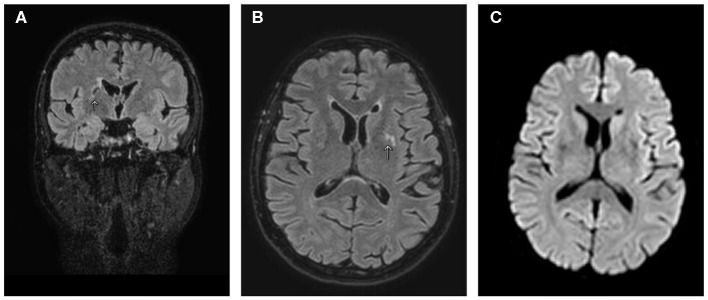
MRI of the brain after 3 months of follow-up. T2/fluid-attenuated inversion recovery (FLAIR) coronal (A) and axial (B) views show size reduction of the pre-existing lesions in the basal ganglia, thalamus, and internal capsule. (C) Diffusion-weighted imaging axial view showing no signal alteration. Arrows indicate CNS lesions.
